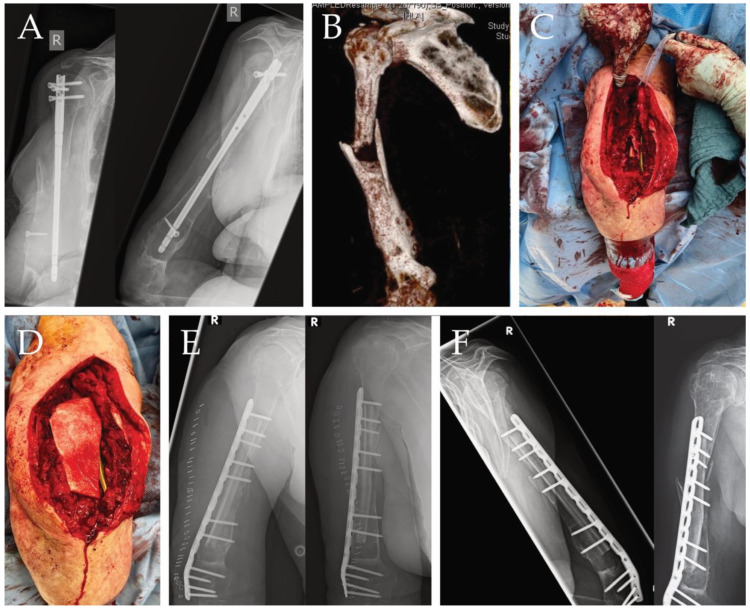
(A) X-ray image of dislocated humerus nail with the large defect zone clearly visible in two planes. (B) CT imaging after nail removal and sampling. (C) intraoperative situs, large defect area clearly visible. (D) operative situs after plate application and installation of a fibula graph as well as defect coverage by a collagen hADM membrane. (E) postoperative imaging, defect completely bridged. (F) healing after six months, clear bony overgrowth of the large defect zone.
